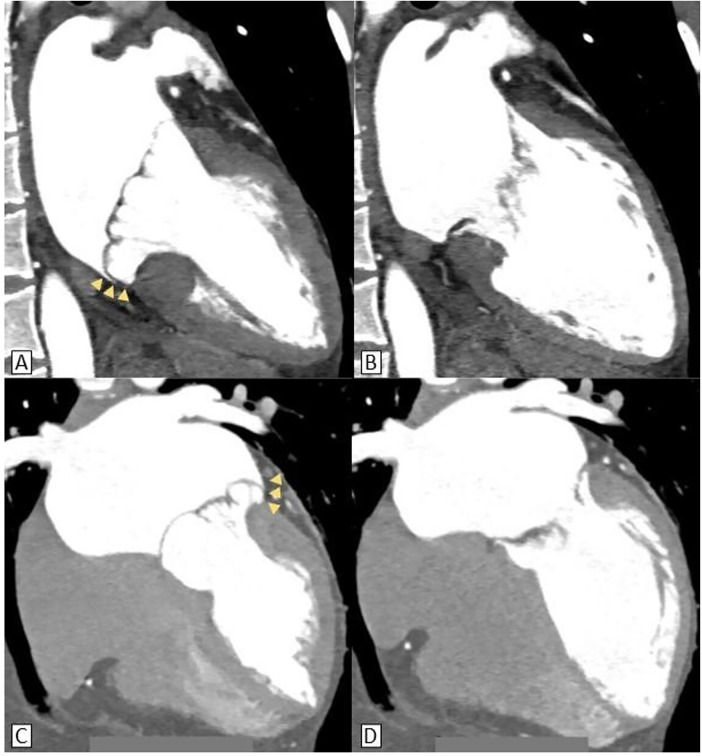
Cardiac computed tomography (CT). The figures show myxomatous mitral leaflets in four- and two-chamber views during systole and diastole (A–D). CT offers high spatial resolution and multiplanar visualization of the mitral valve apparatus, revealing systolic disjunction (A,C), characterized by separation of the posterior mitral leaflet hinge point from the underlying left ventricular myocardium.
